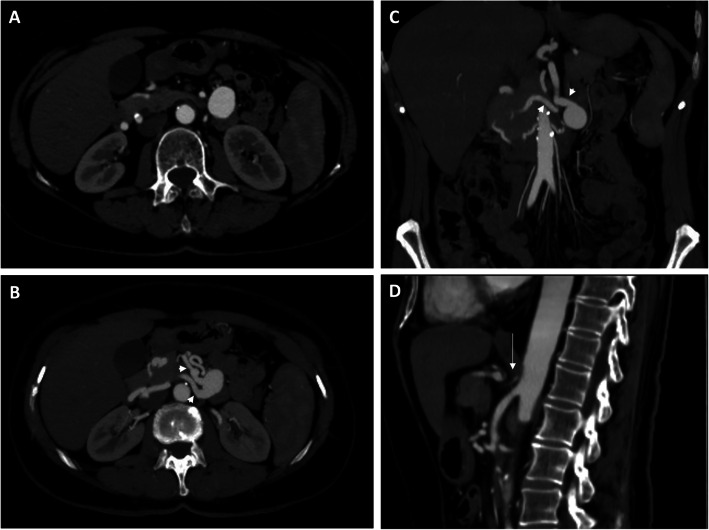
CT angiogram, performed in a 58 years-old woman with a history of abdominal pain and an occasional feedback of an aneurysm of the inferior pancreatic duodenal artery. The aneurysmal sac measured 2,6 × 2,1 cm in maximum diameter (a) (arrowheads). Maximum intensity projected reconstruction (b, c) better depicted the aneurysm morphology with relatively wide neck and dilated inferior pancreatic duodenal artery (arrowheads); the sagittal reconstruction showed celiac trunk occlusion (d, white arrow)
